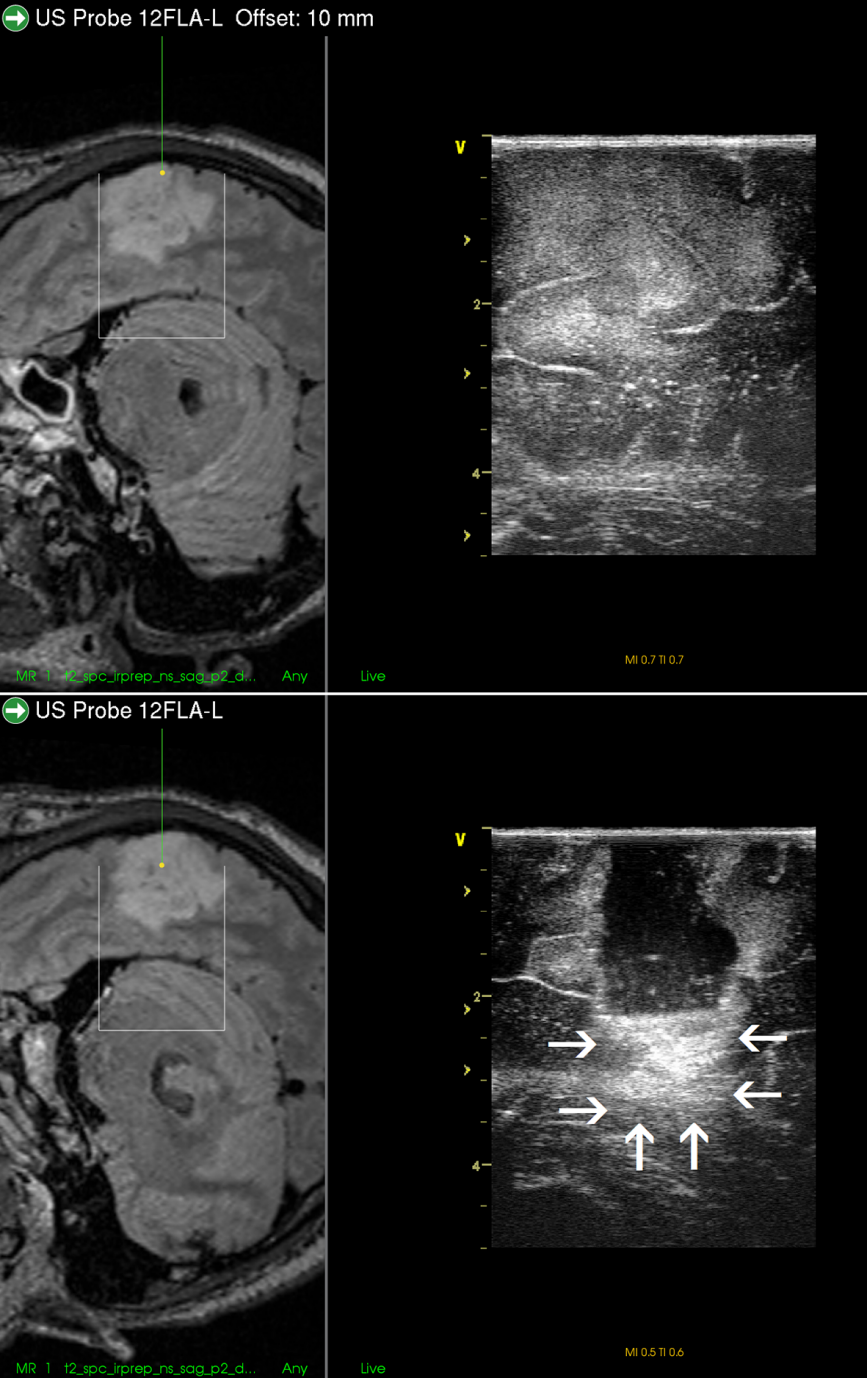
Right-sided temporal grade II oligodendroglioma (Upper row) visualization of the tumor before the resection using 12 MHz linear probe co-registrated with preoperative navigation 3D FLAIR MRI sequence (Lower row) visualization of the tumor after resection of the central tumor part. Note clear visualization of the tumor residua on the sides of the resection cavity, and large acoustic enhancement artifact at the resection cavity-bottom precluding identification of potential tumor remnant in this area. 3D, three-dimensional; FLAIR, fluid attenuated inversion recovery; MRI, magnetic resonance imaging.
